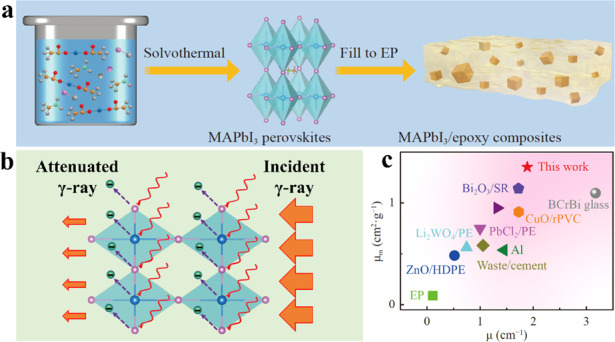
The ionizing radiation, including X-ray and gamma-ray, possesses extremely strong penetration capability, which poses serious risk on the health of the human body due to its damage to deoxyribonucleic acid that would profoundly increase the probability of developing cancer diseases^1,2^. Meanwhile, the ionizing radiation could also jeopardize the efficacy and stability of electronic devices when operated in the environment with high-dose radiation exposure, like in the space or nuclear plants^3–5^. Therefore, the high-performance radiation shielding materials are urgently required to alleviate the radiation risks.
